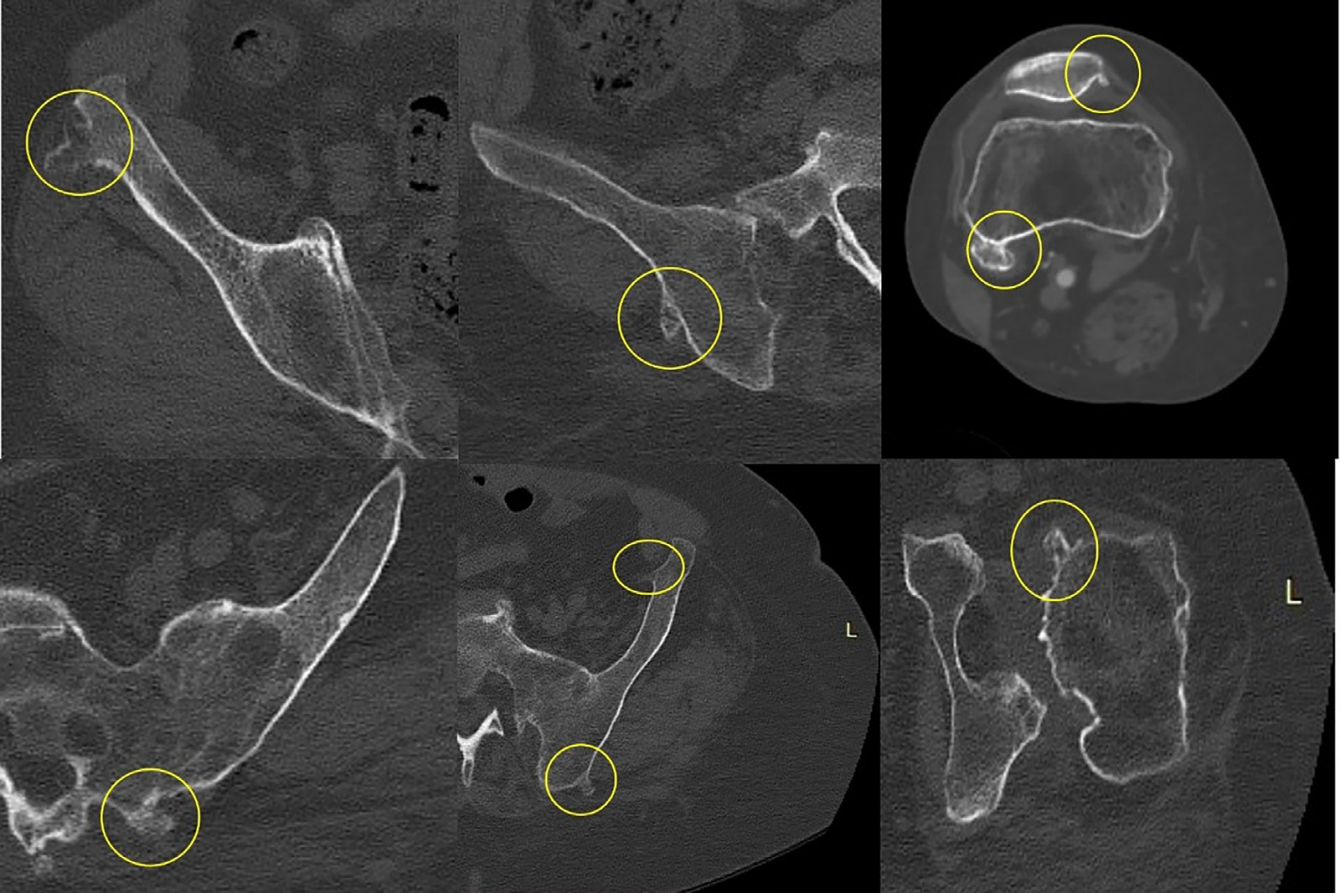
Multiple axial CT scan showing multiple sessile and pedunculated osteochondromas of the pelvis and knee. The bones involved in image order are iliac wing and body on the right, patella and femur on the right, sacral wing on the left, iliac wing and body on the left, femoral head on the left.
